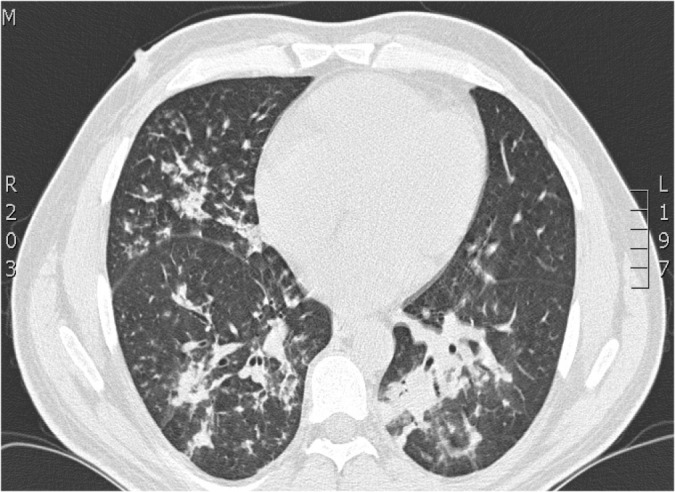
Computed Tomography of the lungs showing multiple nodules and ground glass areas
